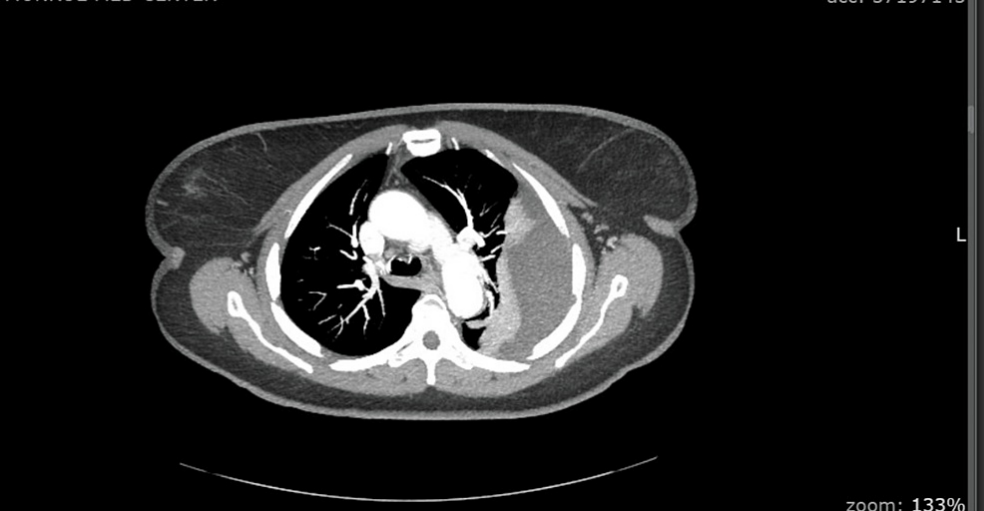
Axial Chest CT With Contrast Showing Large Left-Sided Loculated Pleural Effusion CT, computed tomography
